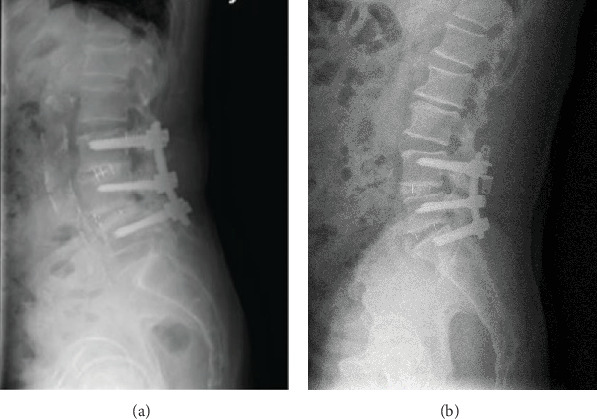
Lateral X-ray of lumbar spine showing (a) PLIF, Pedicle screws were inserted bilaterally at L3–L5 for internal fixation. Interlaminar fenestration or total laminectomy was performed bilaterally at L4/5 for decompression. Interlaminar fenestration was performed bilaterally for decompression at L3/4, with preservation of the lateral 1/2 of the facet joint; (b) hybrid stabilization (Coflex+PLIF), the same procedures were performed to expose the target area and to manipulate the segments at L4–S1 as PLIF. Coflex was inserted to L3/4 with interlaminar fenestration for decompression.
